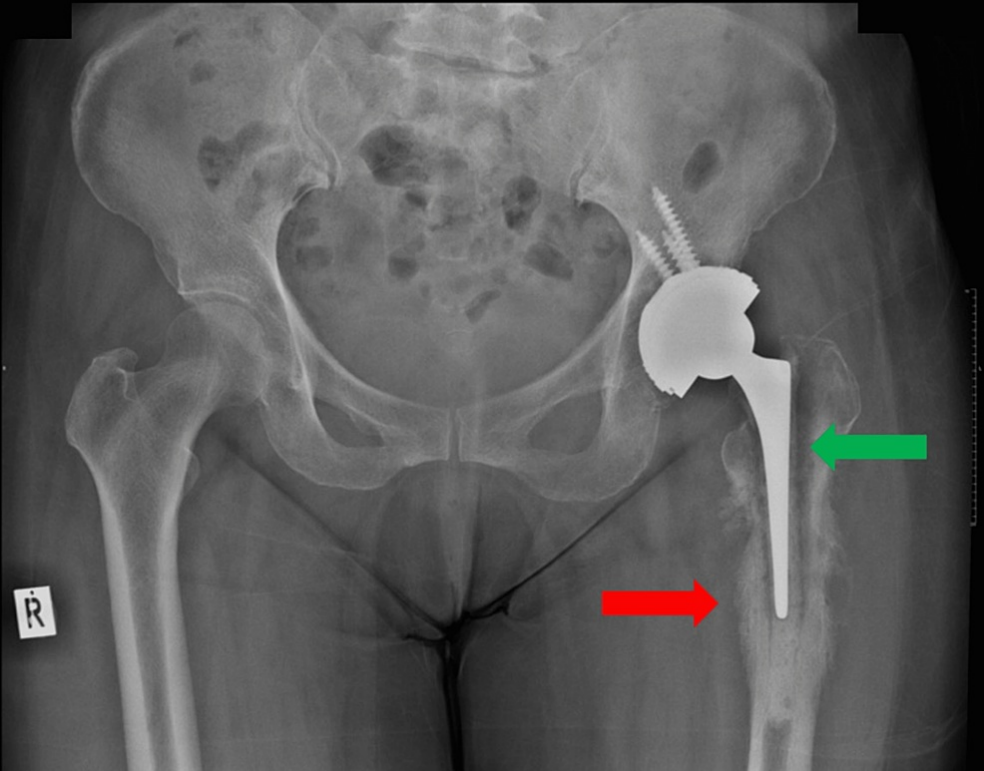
Pelvis AP radiograph showing periosteal reaction at the left proximal femur (red arrow) and loosening of the femoral component (green arrow). AP: anteroposterior
